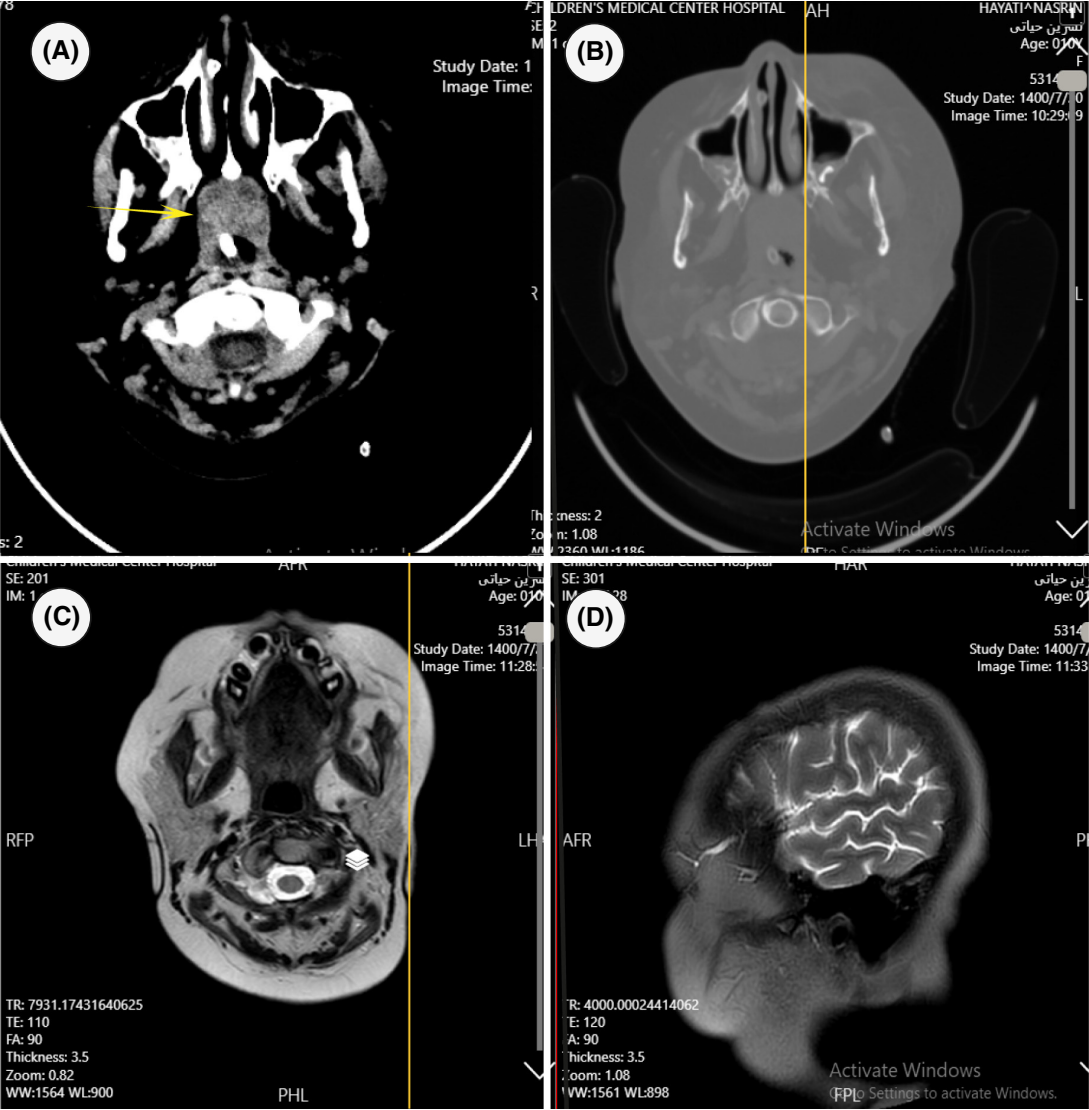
Brain MRI showing two lesions in the right parietal lobe and left frontal lobe (A–D).
